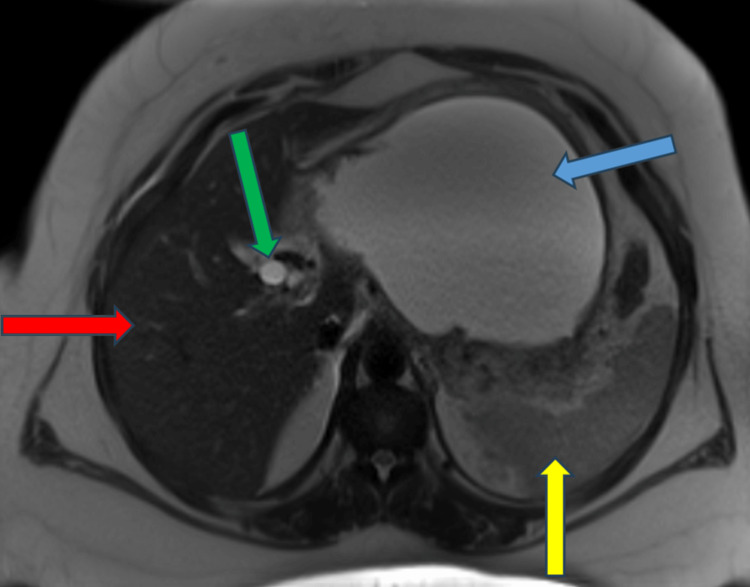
T2-weighted image on MRI scan shows a huge pancreatic pseudocyst (blue arrow); liver (red arrow), spleen (yellow arrow), and dilated CBD (green arrow) can be seen as well. CBD: common bile duct
